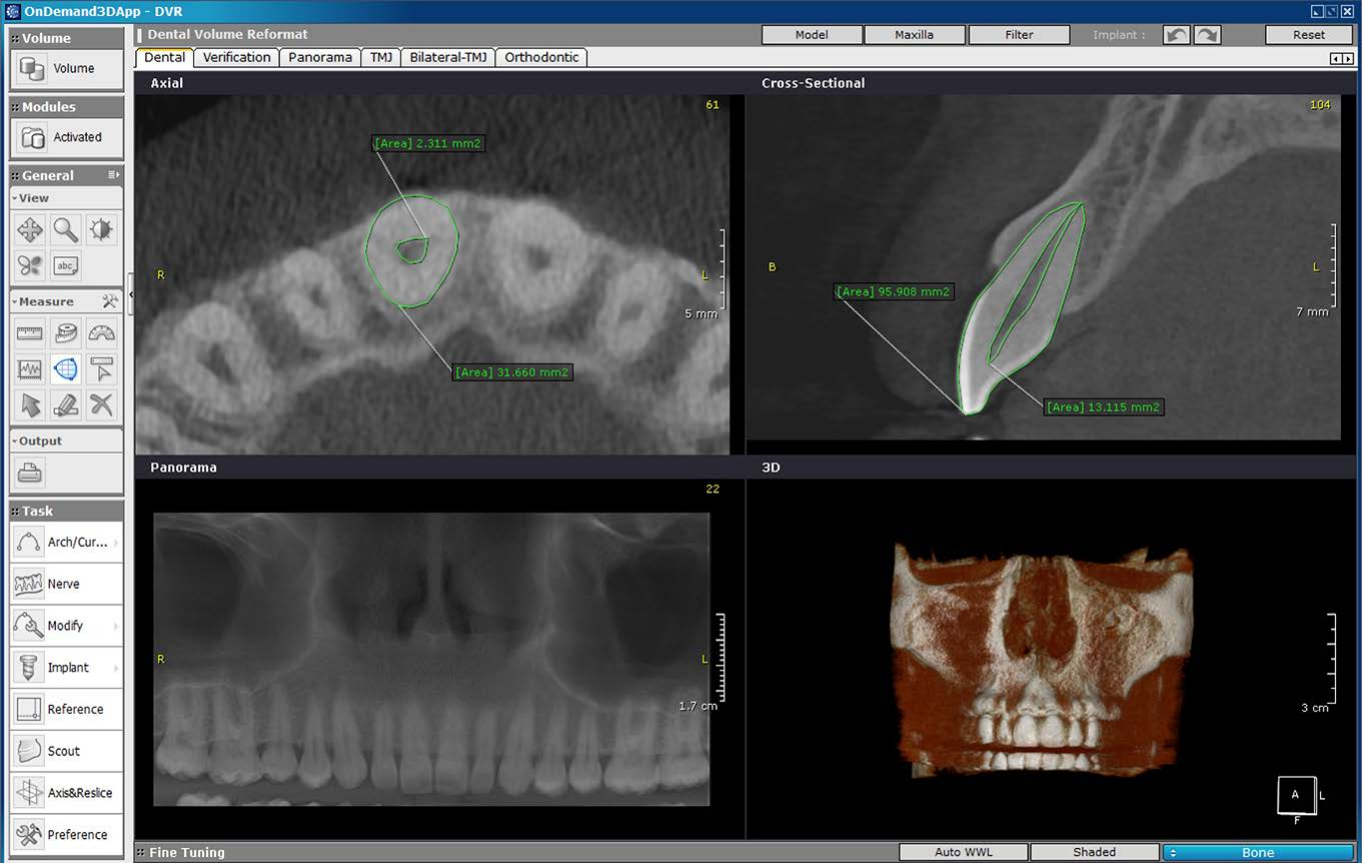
Axial and sagittal views of the maxillary central incisor from CBCT seen in OnDemand 3D Dental program. The outer line delineate the area of the tooth. The inner line delineate the area of the pulp
